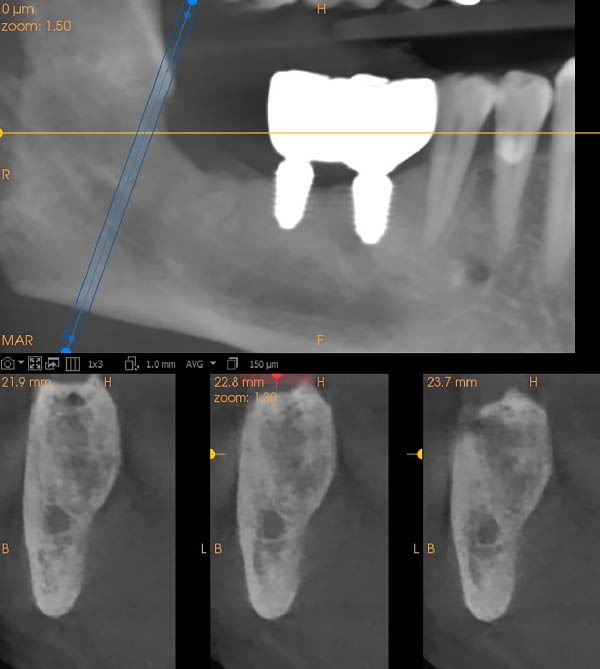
(Top) Cropped reformatted panoramic image of the right mandibular region showing healing in the area of the extracted right mandibular third molar. (Bottom) Buccolingual cross‐sectional CBCT images demonstrate healing and bone formation in the area with intact cortical borders and no sign of recurrence.
